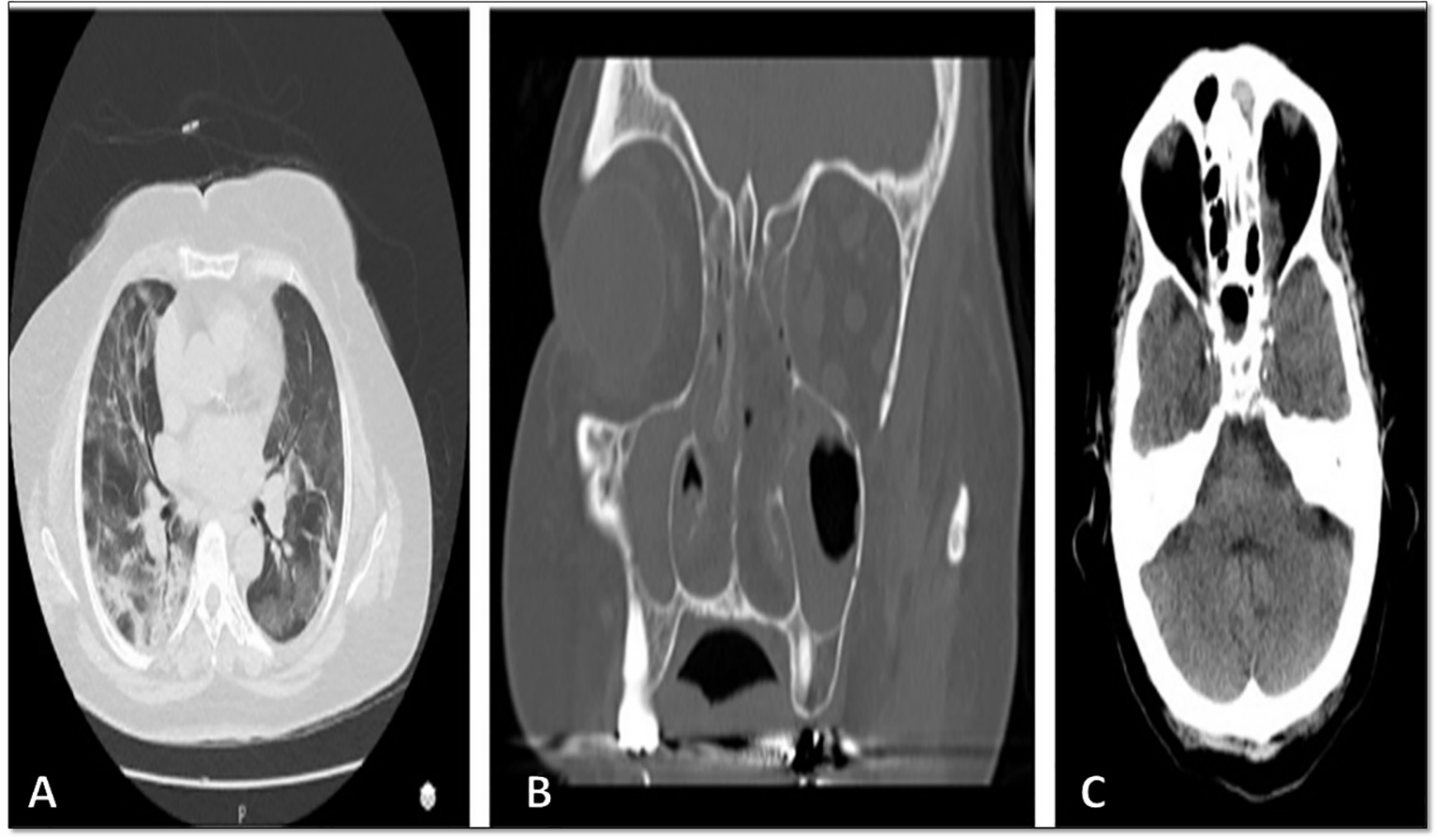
(A) Chest CT scan showing patchy ground-glass opacity, bilateral consolidation, and atelectatic bands. (B, C) Paranasal and orbital CT scan displaying opacification in all paranasal sinuses, destruction of medial septum in the left maxilla, bilateral retrolental fat haziness, medial extra and intraconal fat haziness, and inflammation of medial and superior rectus muscles. These findings suggest a fungal sinonasal invasion in the right orbital.
